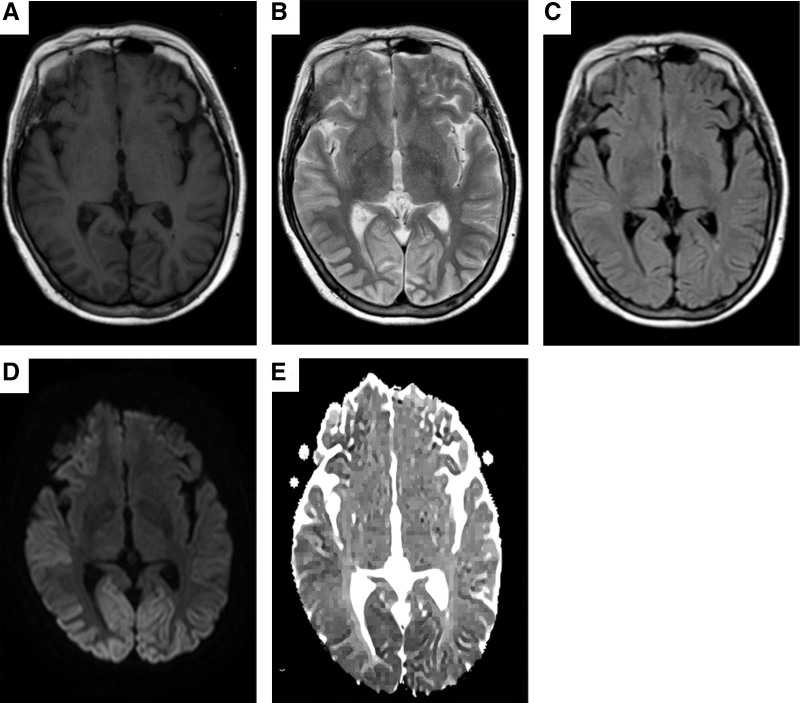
Cranial MRI of case 3. The cranial MRI of the 3rd patient is shown in A to E. (A) Transverse axial T1WI showed no obvious abnormal signal. (B) Transverse axial T2WI showed high signal in bilateral occipital, right frontal and temporal lobes. (C) Transverse axial FLAIR shows slightly high signal in the right temporal lobe. (D) Transverse axial DWI sequence showing high signal in the bilateral occipital, right frontal and temporal lobes, typical of ribbon sign. (E): ADC shows low signal in the area corresponding to the DWI. DWI = diffusion-weighted imaging, FLAIR = fluid-attenuated inversion recovery.
